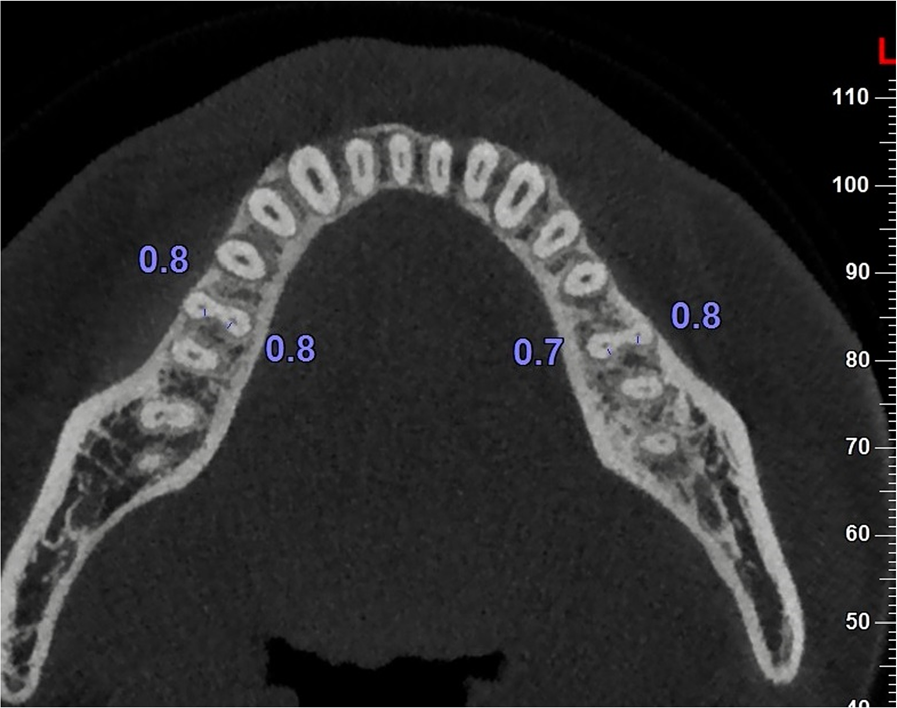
Measurement of the minimum distal dentine thickness of danger zone on CBCT images
